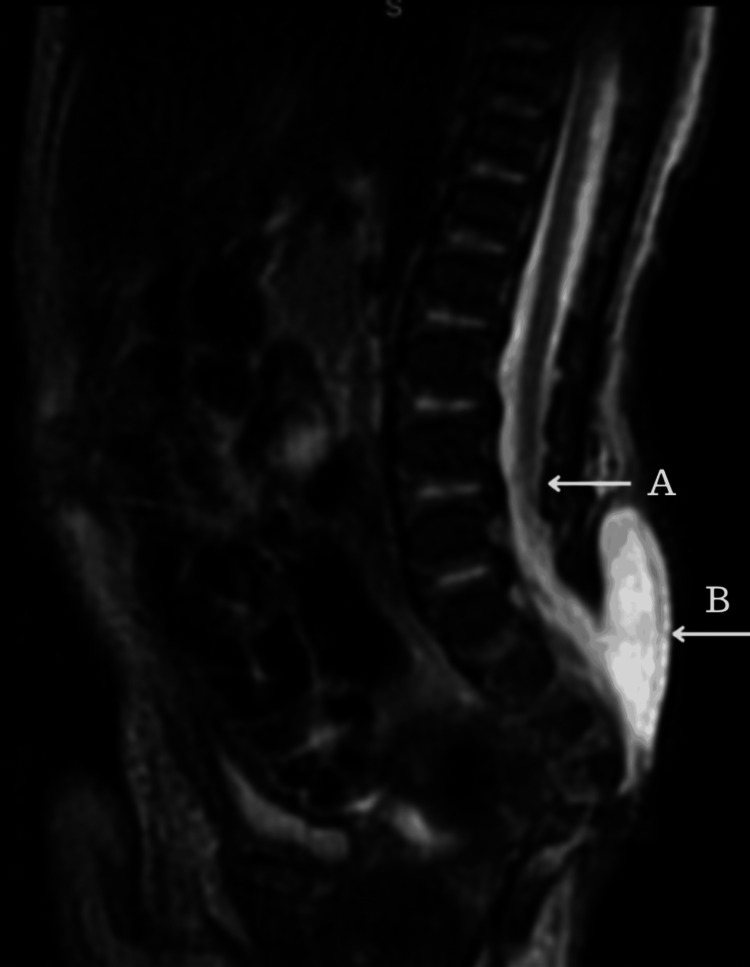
Lumbosacral spine MRI sagittal T2-weighted image demonstrates a tethered spinal cord (Arrow A) down to the meningomyelocele that is herniated through a large sacral bony defect (Arrow B).
